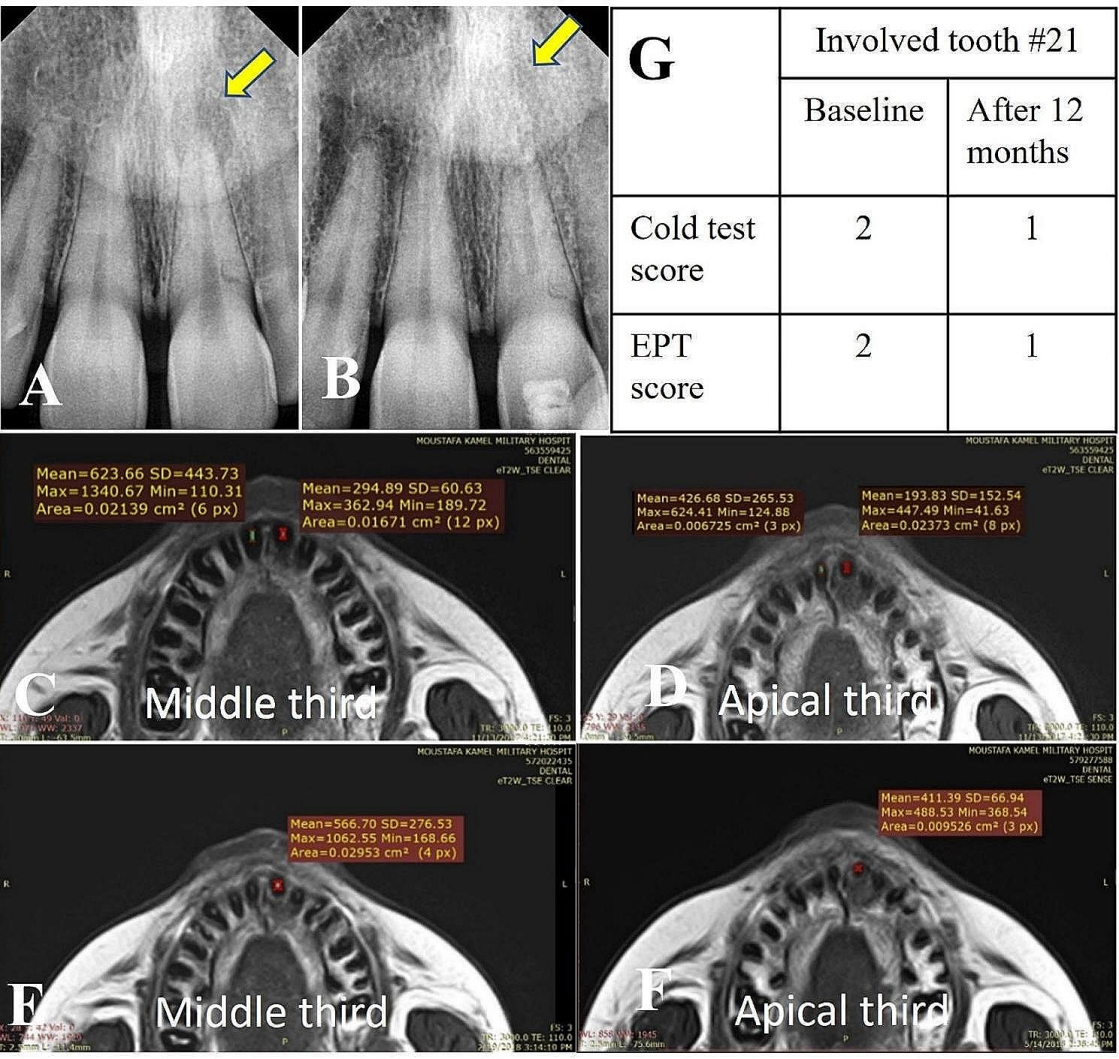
Radiographic images of a representative case: (A) Digital preoperative radiographs with periapical lesions related to tooth #21 (yellow arrow). (B) After 12 months follow-up showing periapical healing (yellow arrow). (C)(D)(E)(F) MRI signal intensity measurements (C) and (D) Middle and apical thirds baseline for both involved tooth (#21) and normal contralateral (#11) (E) (F) Middle and apical thirds after 12 months follow-up period. (G) table representing the cold test and EPT scores for the involved tooth #21
